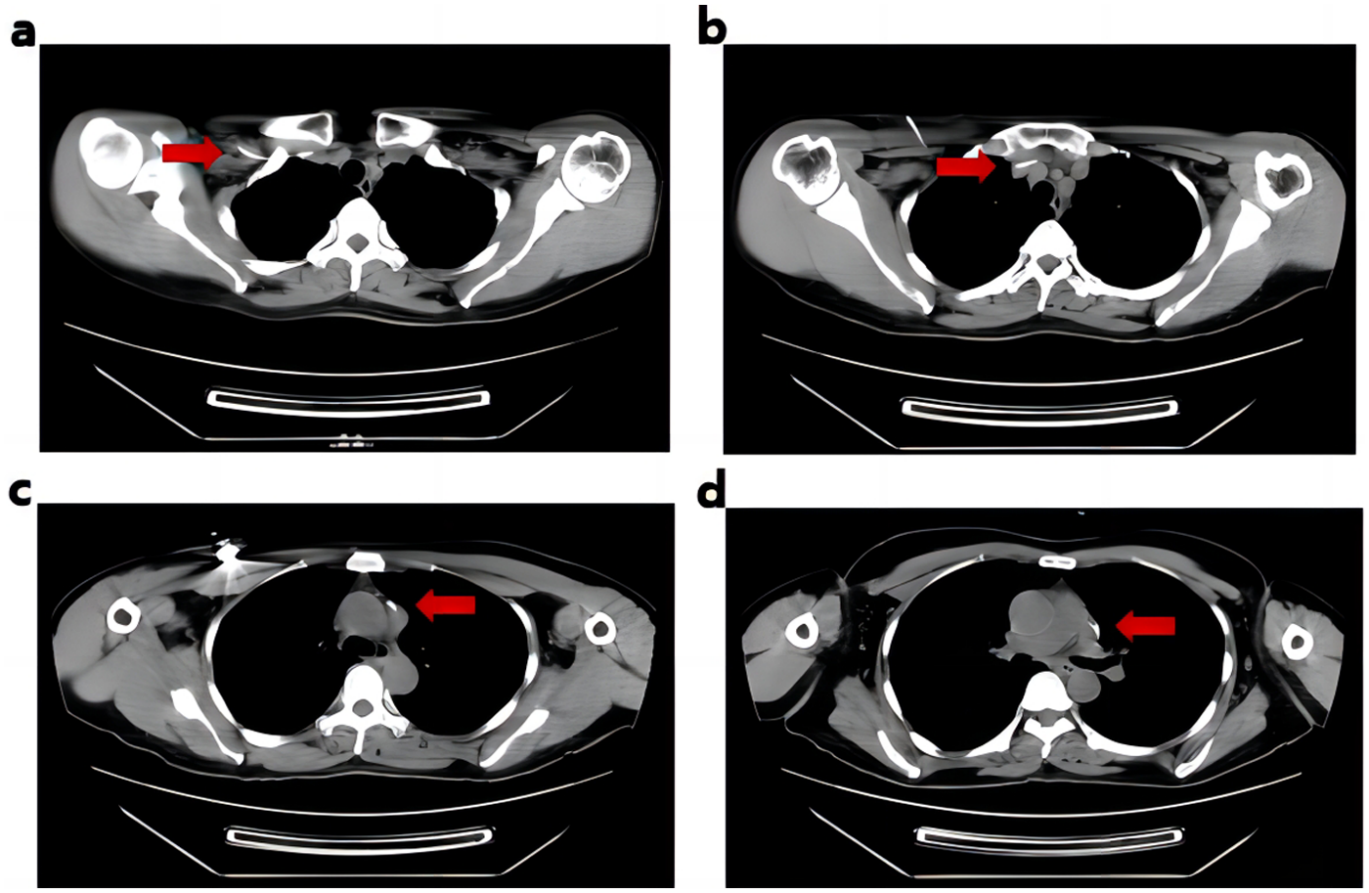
Postoperative CT showed the position of the catheter. (a) The catheter was located under the right subclavicle. (b) The catheter was inserted into the innominate vein. (c) The catheter was entered into the left superior vena cava. (d) The tip of the catheter was located at the left lateral level of the lower border of the 6th thoracic vertebra, within the middle and lower 1/3 of the left persistent superior vena cava.
